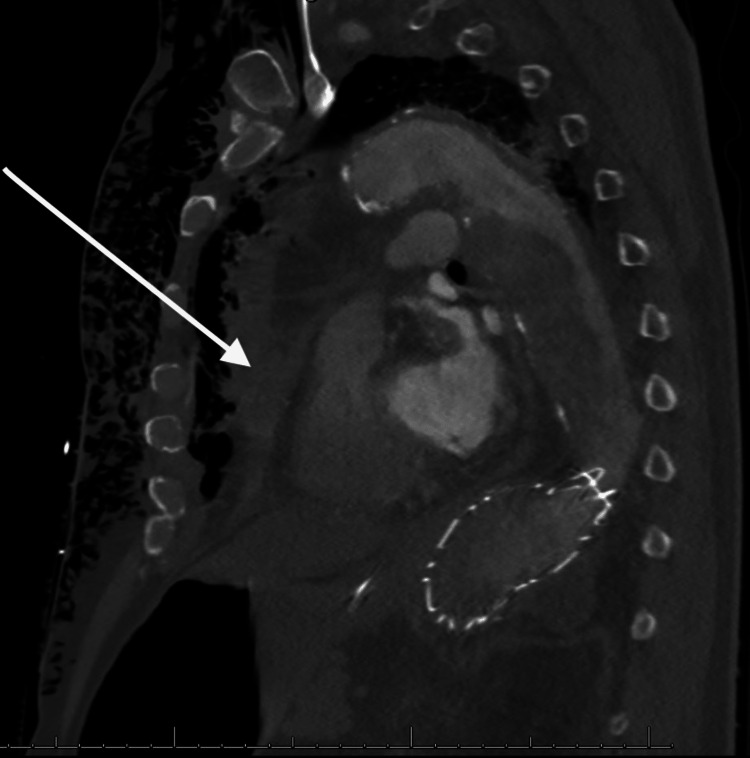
CT scan of the chest sagittal view showing hemopericardium (see white arrow)
